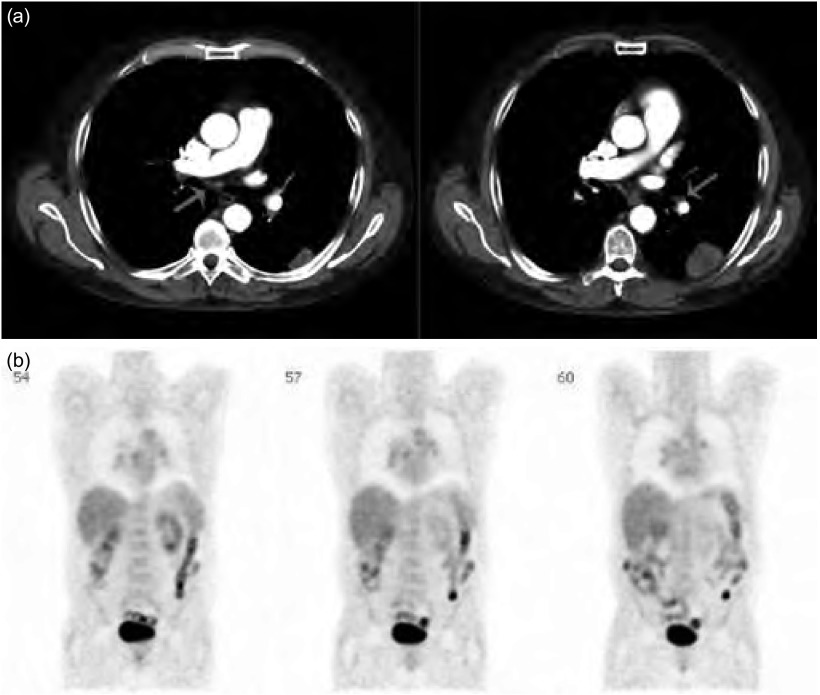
例5。（a）：胸部CT图像；（b）：PET图像显示外周肿块、多方肺门、纵膈淋巴结阳性。 Case 5. (a) CT chest. (b) Case 5 PET scan showing peripheral mass as well as multiple symmetrical hilar and mediastinal lymph node positivity.
